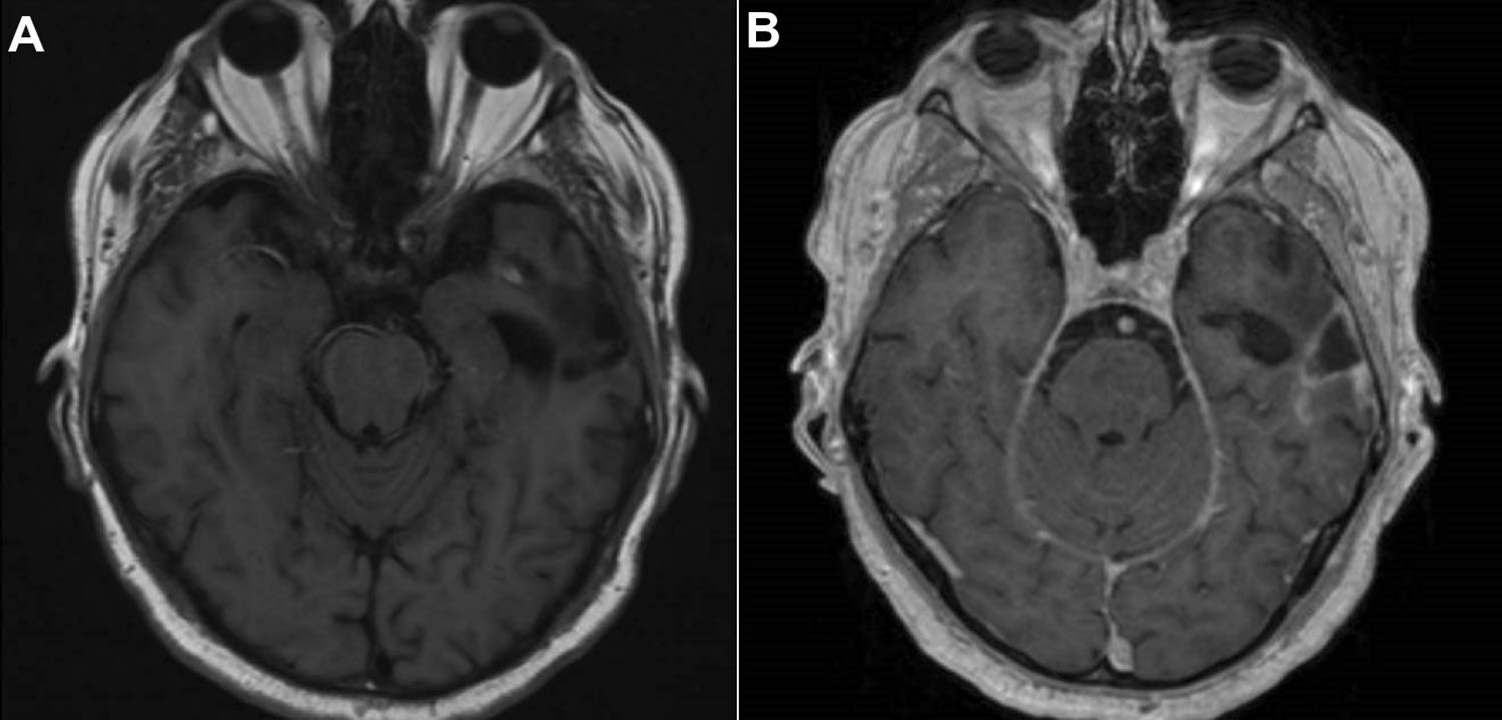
MRI at 3 months follow-up. The transverse view (A, T1 weighted image, not contrast enhanced; B, contrast-enhanced T1 weighted image) shows the area of the prior cystic lesion with a regressive perifocal edema
